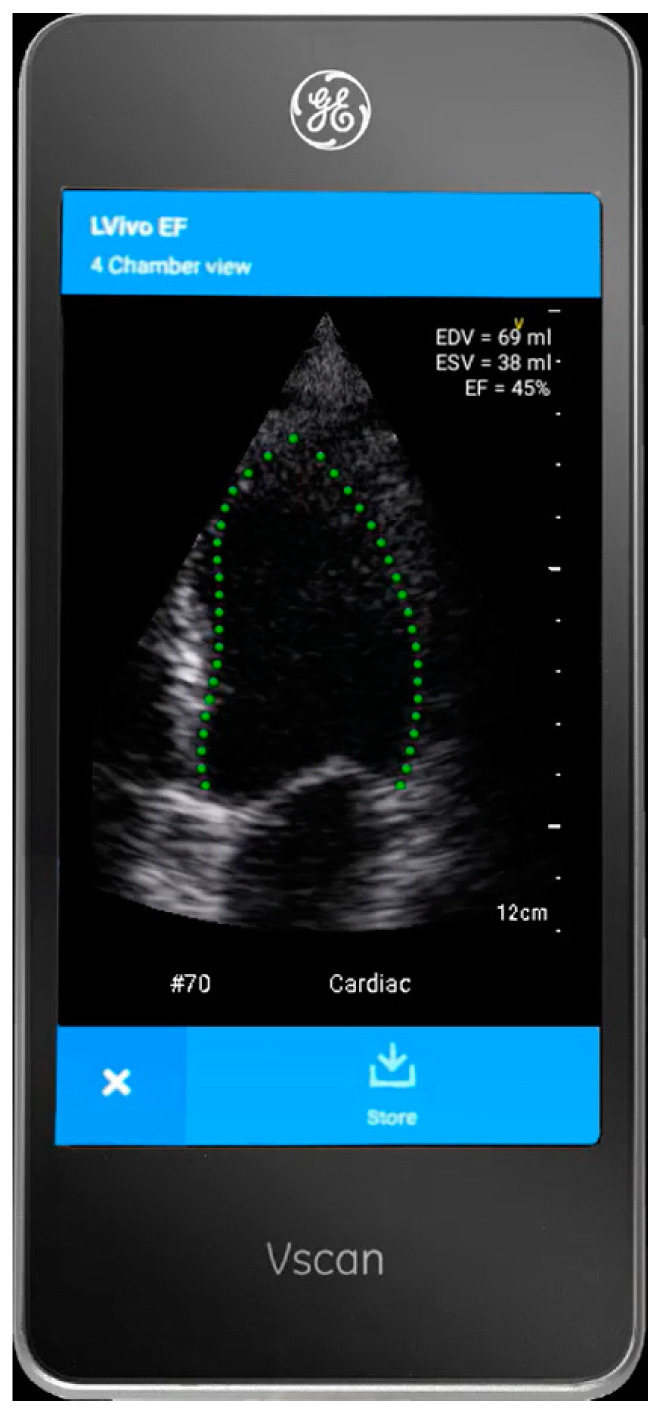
LVivo EF, I AI tool for automatic LVEF evaluation from the A4ch view using the Vscan Extend HUD. Abbreviations: A4ch, apical 4-chamber; AI, artificial intelligence; HUD, hand-held ultrasound device; LVEF, left ventricular ejection fraction.
